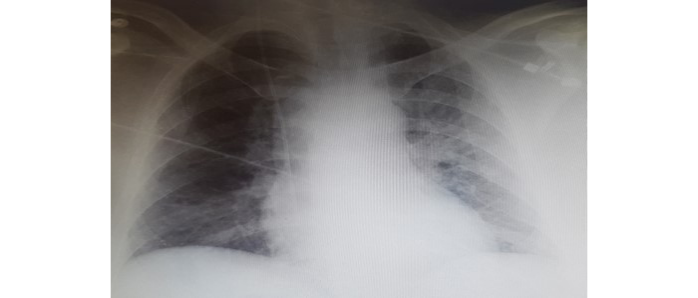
Thoracic radiography of the first patient. Mitral regurgitation associated with left ventricular outflow tract obstruction is most often eccentric and travels to the left pulmonary veins, resulting in unilateral acute pulmonary edema in this patient.
